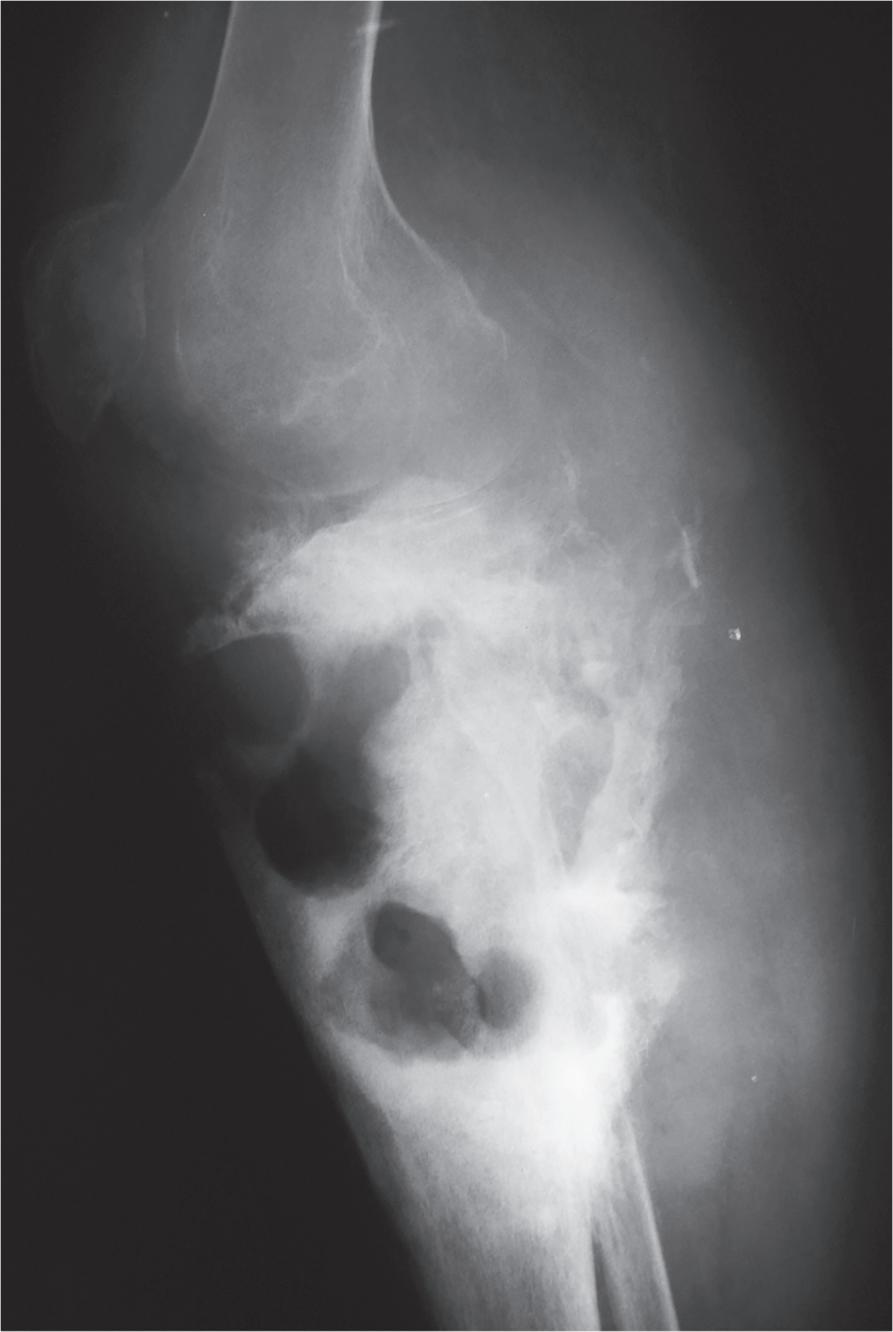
Showing knee region X-ray with type finding of eumycetoma; soft tissue mass, periosteal reaction of the lower part of the femur, patella and upper tibia and multiple bone cavities in the upper part of the tibia.
